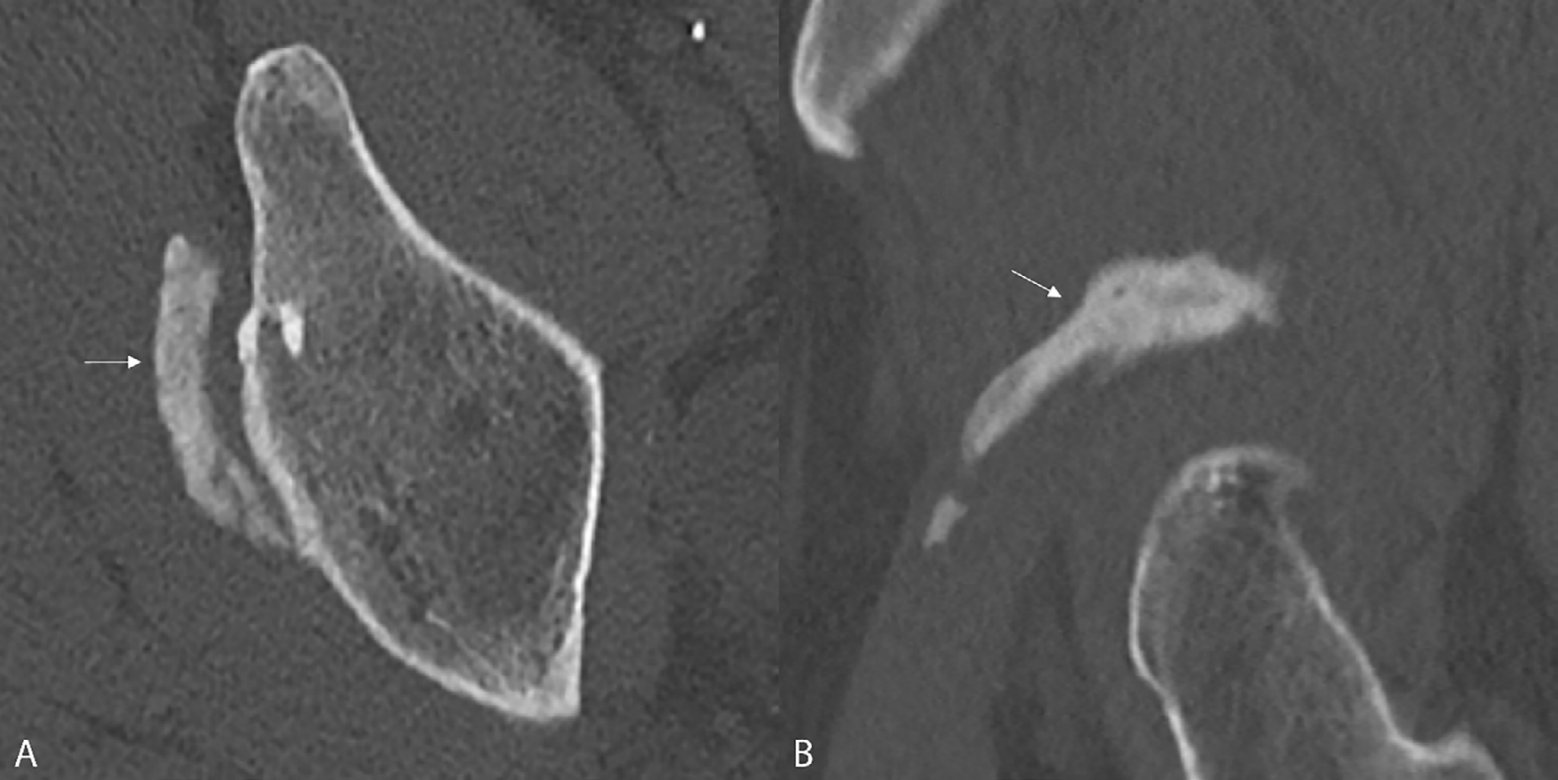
Computed Tomography confirms HO with elongated morphology superior to the acetabular rim (arrows).
